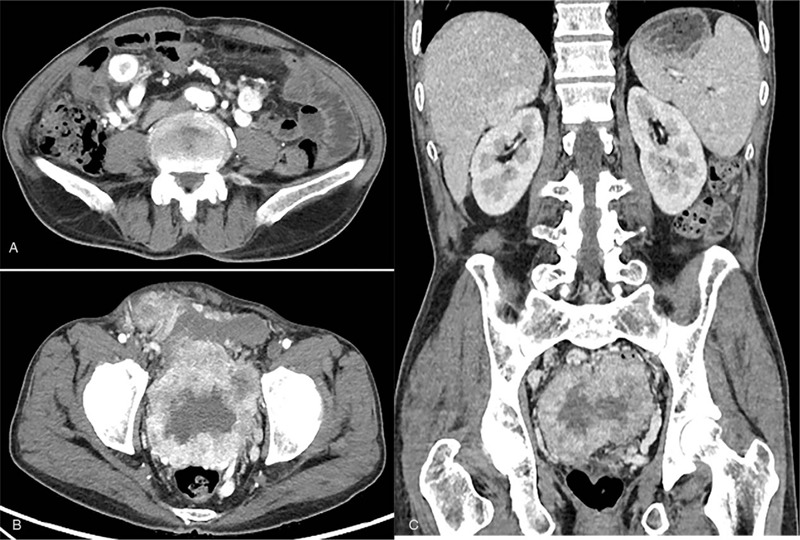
CT enhancement and 3D reconstruction imaging. (A) Multiple nodules in the abdominal cavity. (B) A huge tumor in the pelvic cavity. (C) Inhomogeneous strengthening.
